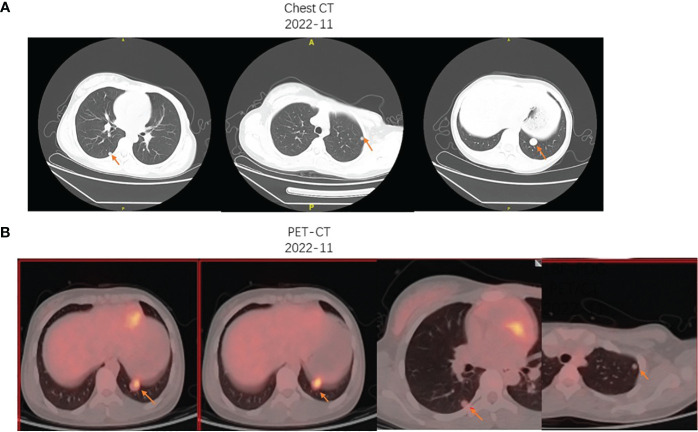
(A) The chest CT imaging presented the multiple pulmonary nodules in the right and left lung, with the biggest metastasis measuring nearly 2 cm. (B) The 18F-FDG PET/CT showed an increased FDG uptake of the largest left pulmonary nodule (inferior lobe; SUVmax, 3.9) and mild FDG uptake of the multiple pulmonary nodules.
